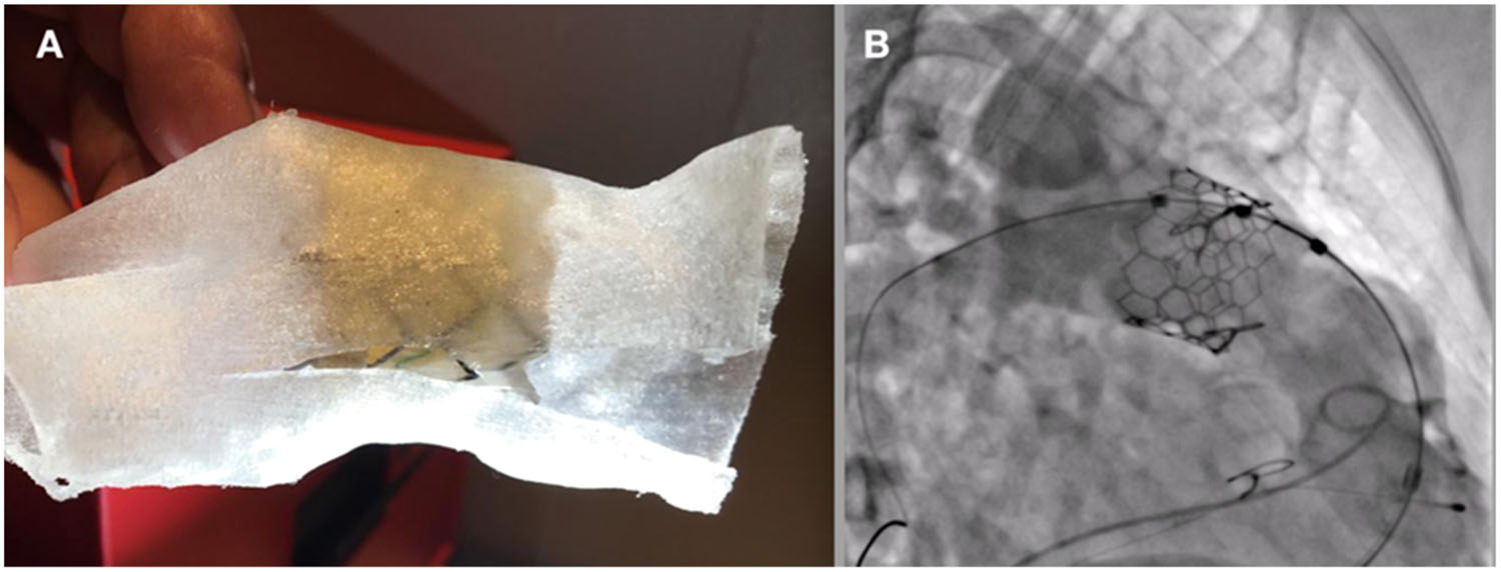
Pre‐implantation computed tomography with three‐dimensional reconstruction and bench test with “in vitro” implantation of a 32‐mm‐diameter valve at the supra‐annular position (A) and post‐implant supra‐annular position with final adequate result (B).
